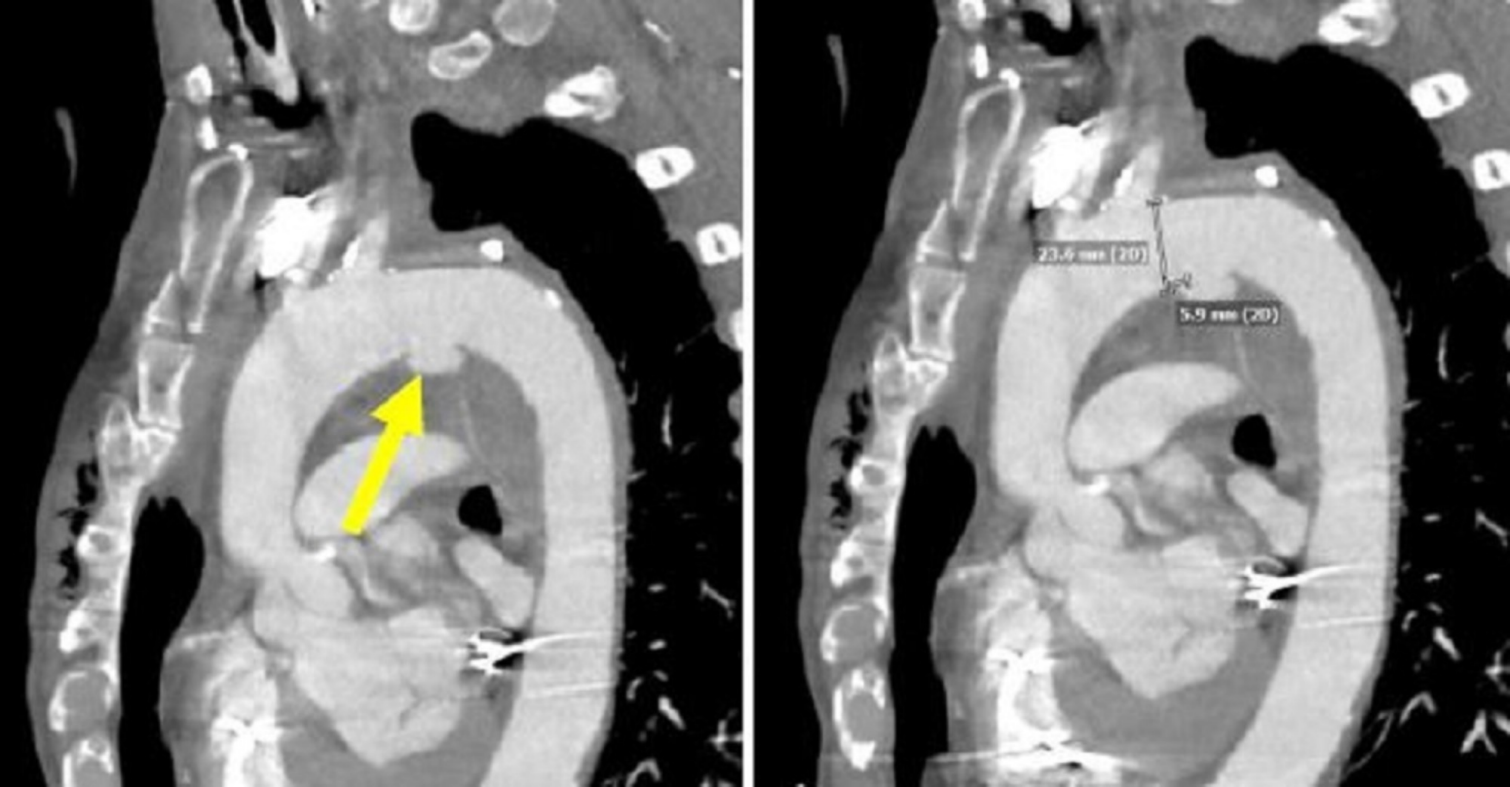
Sagittal computed tomography angiography on the left showing a traumatic pseudoaneurysm (yellow arrow). The image on the right shows the caliper along the lesser curve measuring 5.9 mm as a proximal landing zone neck.
